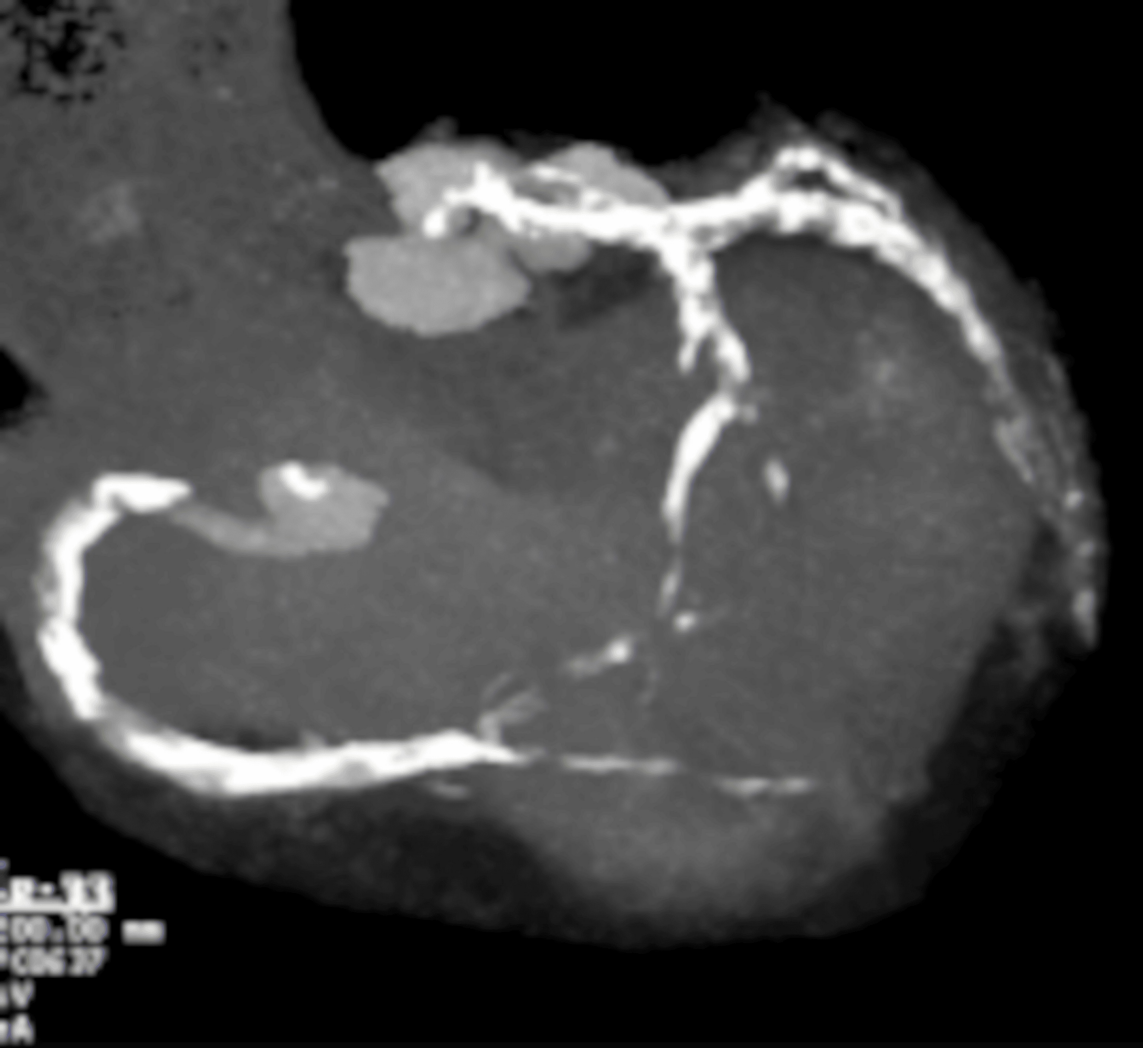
Coronary computed tomography angiography showing severe calcification in the right and left coronary arteries.
